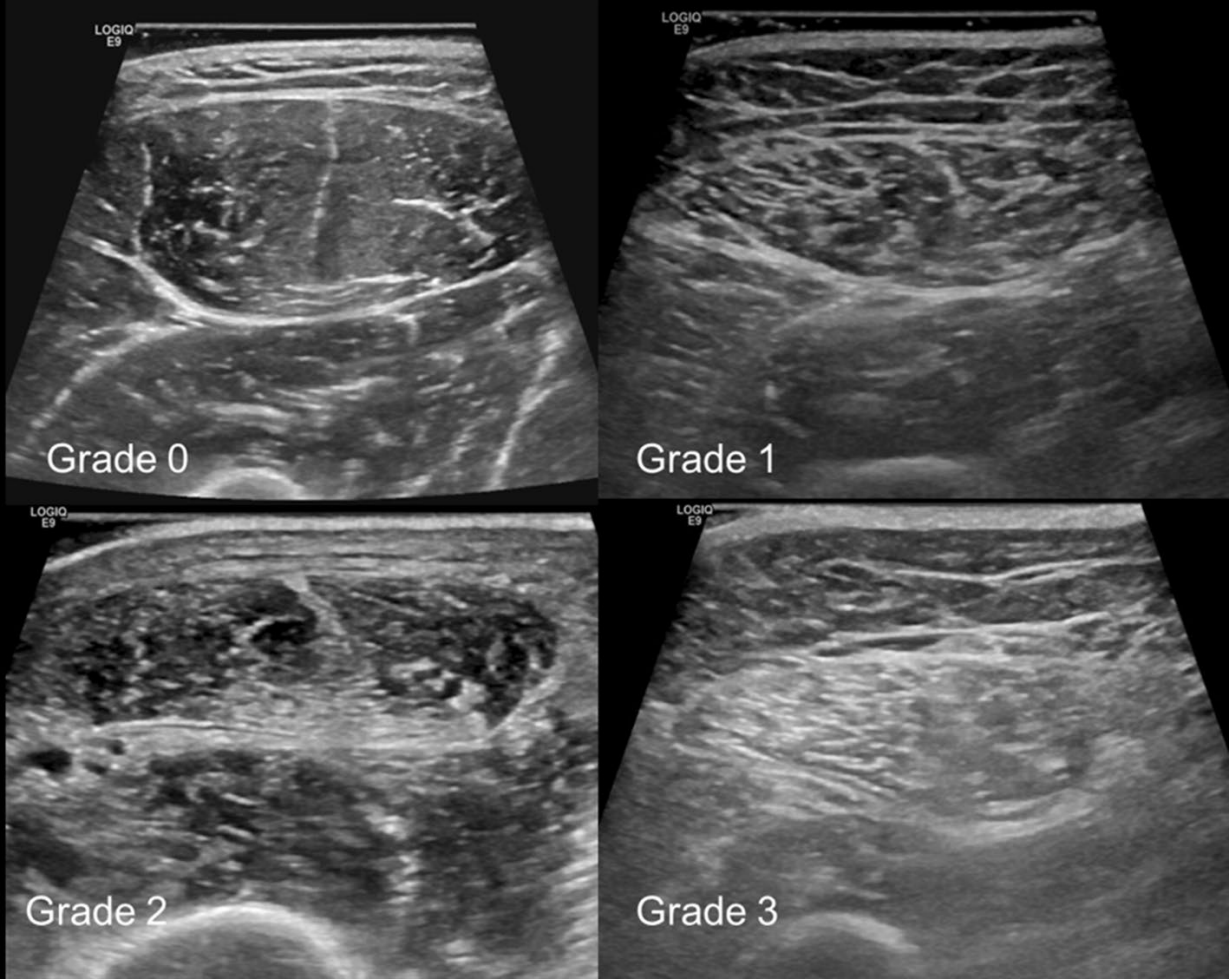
Muscle echogenicity grading on gray-scale ultrasonography of right mid rectus femoris muscle.
